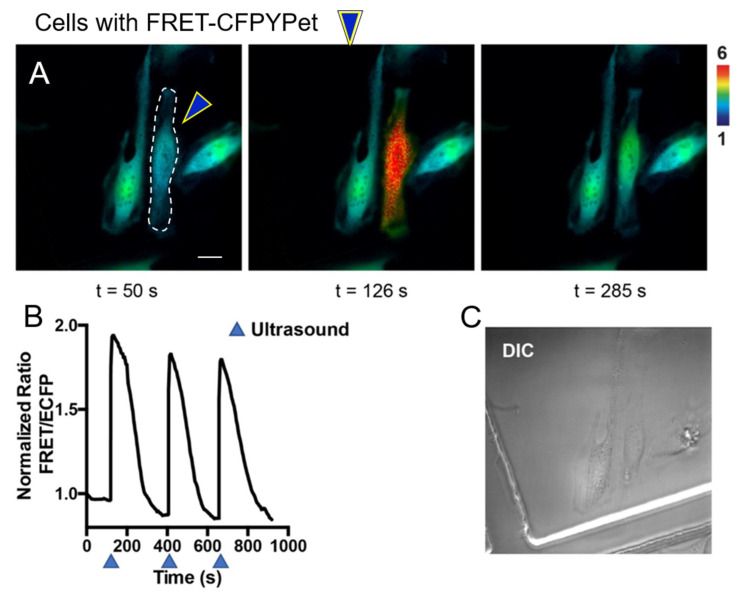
Repeated and transient HFU stimulation. (A) Images and (B) time course of FRET/ECFP signal ratio of Ca^2+^ biosensor show transient and repeated stimulations by high-frequency ultrasound at the target single cell (dashed line in A). (B) High-frequency ultrasound (arrowheads) was applied three times for 5 μs. (C) A DIC image of cells in (A).
